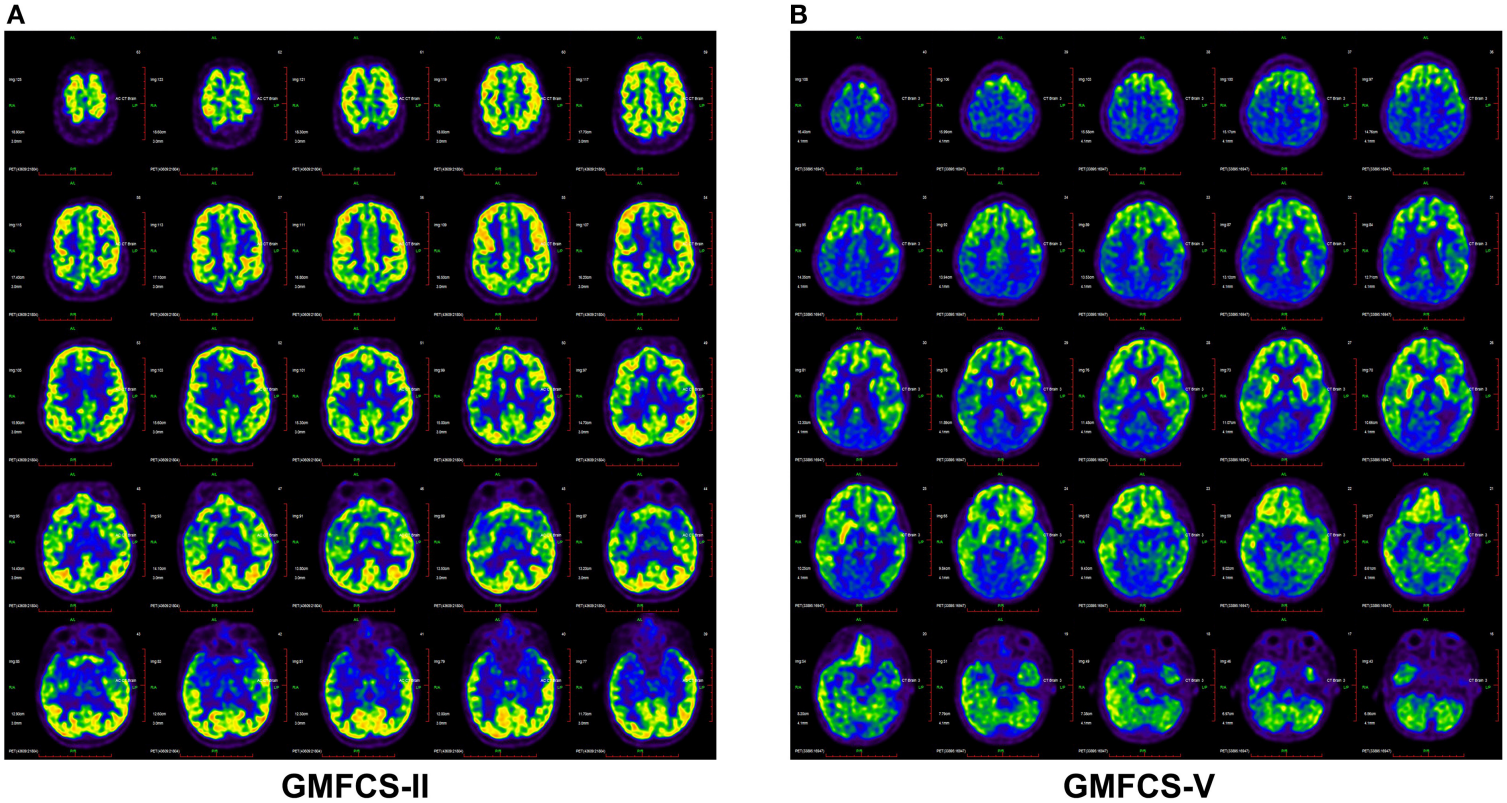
Brain ^18^F-FDG PET images demonstrated different metabolic activity of CP patients with GMFC level II (A) and V (B). The CP patients with GMFC level V exhibited decreased activity compared with GMFC level II.
